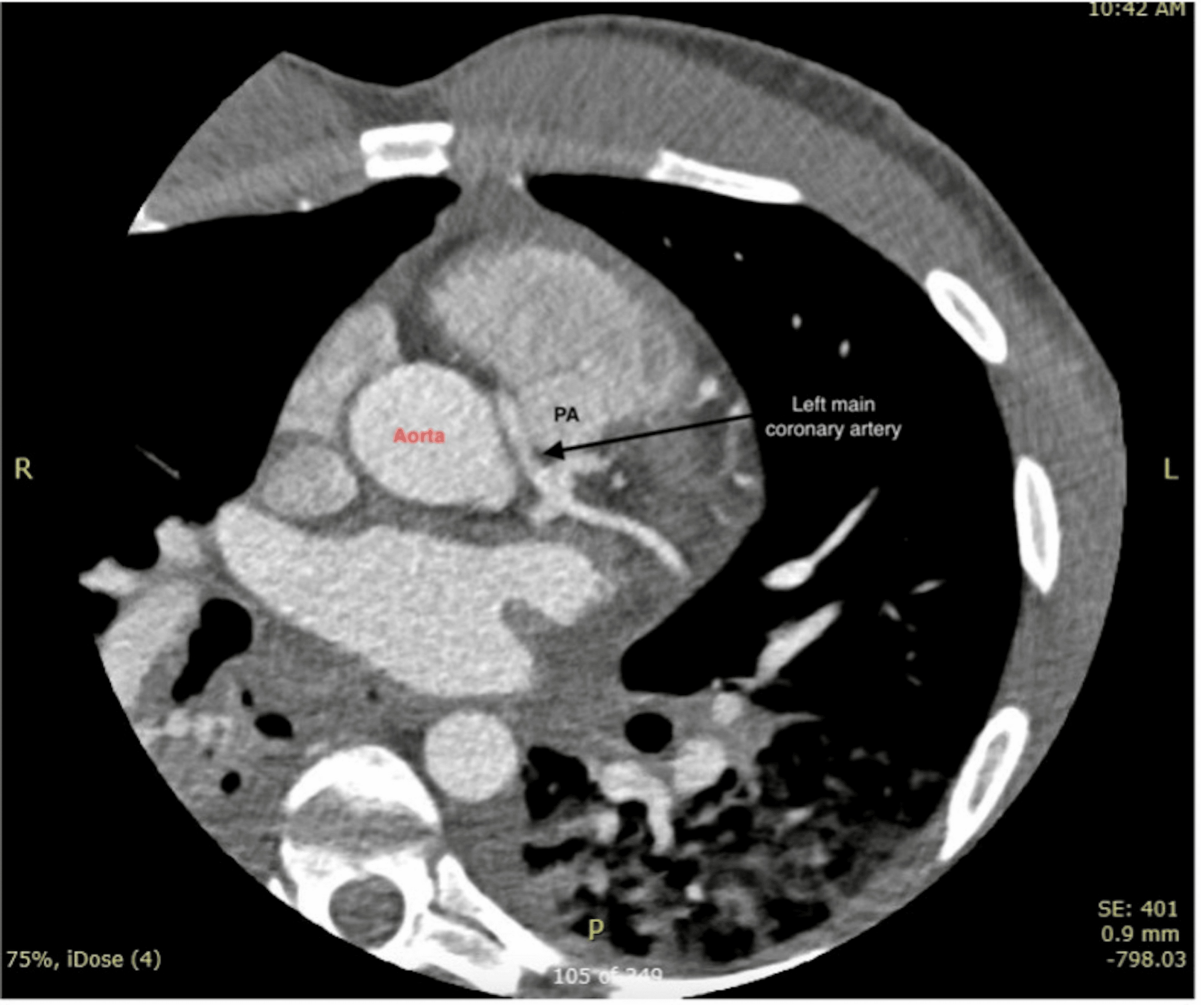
CCTA with arrows revealing the patient’s anomalous left main coronary artery coursing between the ascending aorta and main pulmonary artery. CCTA, coronary computed tomography angiography; PA, pulmonary artery
